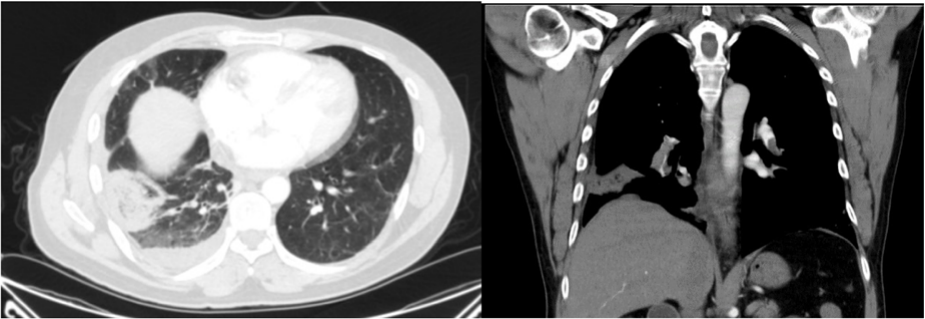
Pulmonary embolism: thin section CT in axial plane in lung window shows subpleural consolidation with reverse halo sign(RHS), coronal image in mediastinal window show filling defect in lower lobes pulmonary arteries suggestive for pulmonary embolism
